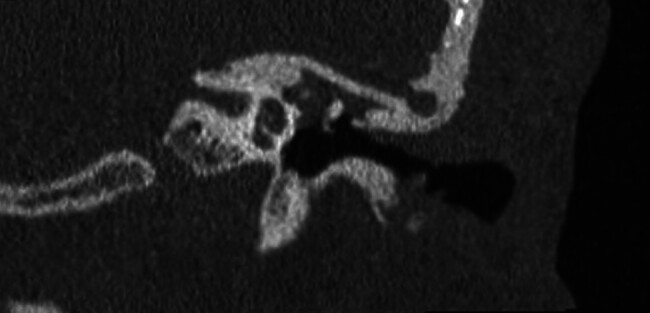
Postoperative computed tomography showing the “manhole cover” method of covering the tegmen defect.
